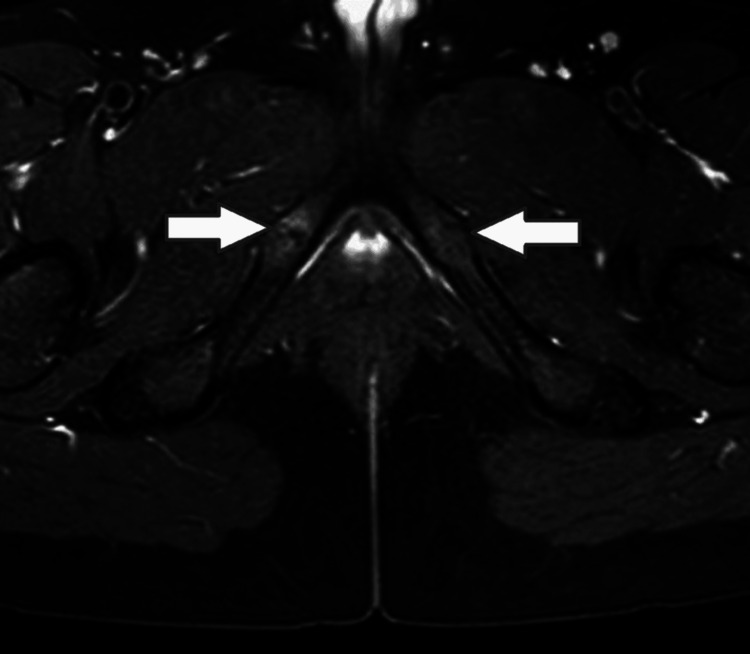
MRI showing an increased intensity with a fibrous band surrounded by an edematous change in the area of the bilateral ischiopubic synchondroses.
